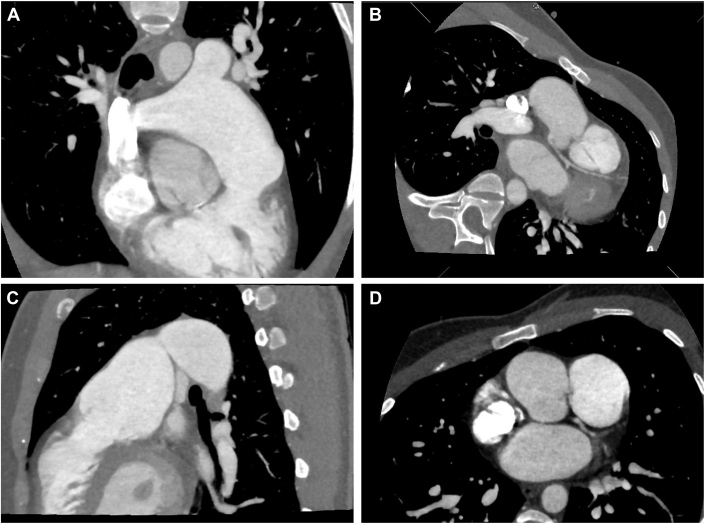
Cardiac Computed Tomography Planning Prior to the Procedure Three-dimensional construction of the RVOT and the pulmonary annulus showing different measurements in systole and the proximity of the pulmonary annulus to the left anterior descending artery (LAD) (a-e). RVOT = right ventricular outflow tract.
